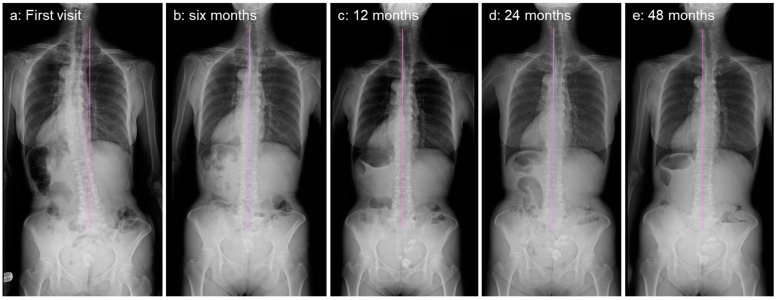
Standing antero-posterior whole-spine radiographs at first visit (a), 6 months (b), 12 months (c), 24 months (d), and 48 months (e). Straight vertical line indicates CSVL.
